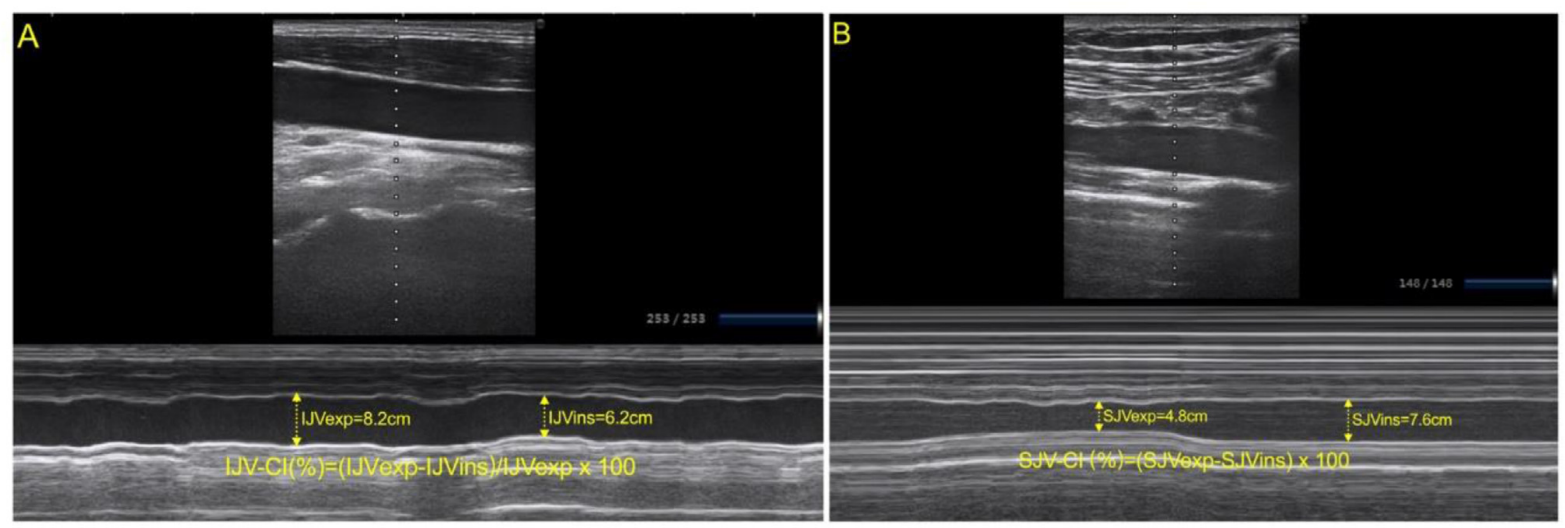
Example ultrasound images of IJV-CI, IJV-SV, and SCV-CI. IJV-CI (A) was measured at the level of the cricoid cartilage and calculated by IJV-CI (%) = (IJVexp-IJVins)/IJVexp × 100 [8]. SCV-CI (B) were measured perpendicular to the clavicle long axis and calculated by SCV-CI(%) = (SCVexp-SCVins)/SCVexp× 100 [9]. IJV-CI: the respiratory variability in the diameter of internal jugular vein; SCV-CI: the respiratory variability in the diameter of subclavian vein.
